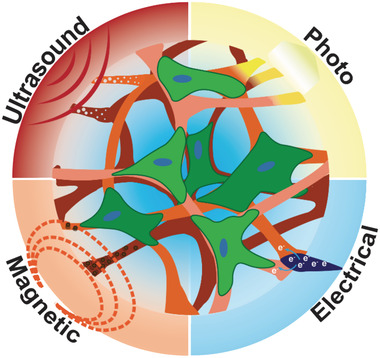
Stimuli‐responsive scaffolds transduce exogenously applied signals to stimulate stem cells. These externally applied stimuli can include light, electrical stimulation, ultrasound, and magnetic fields.
